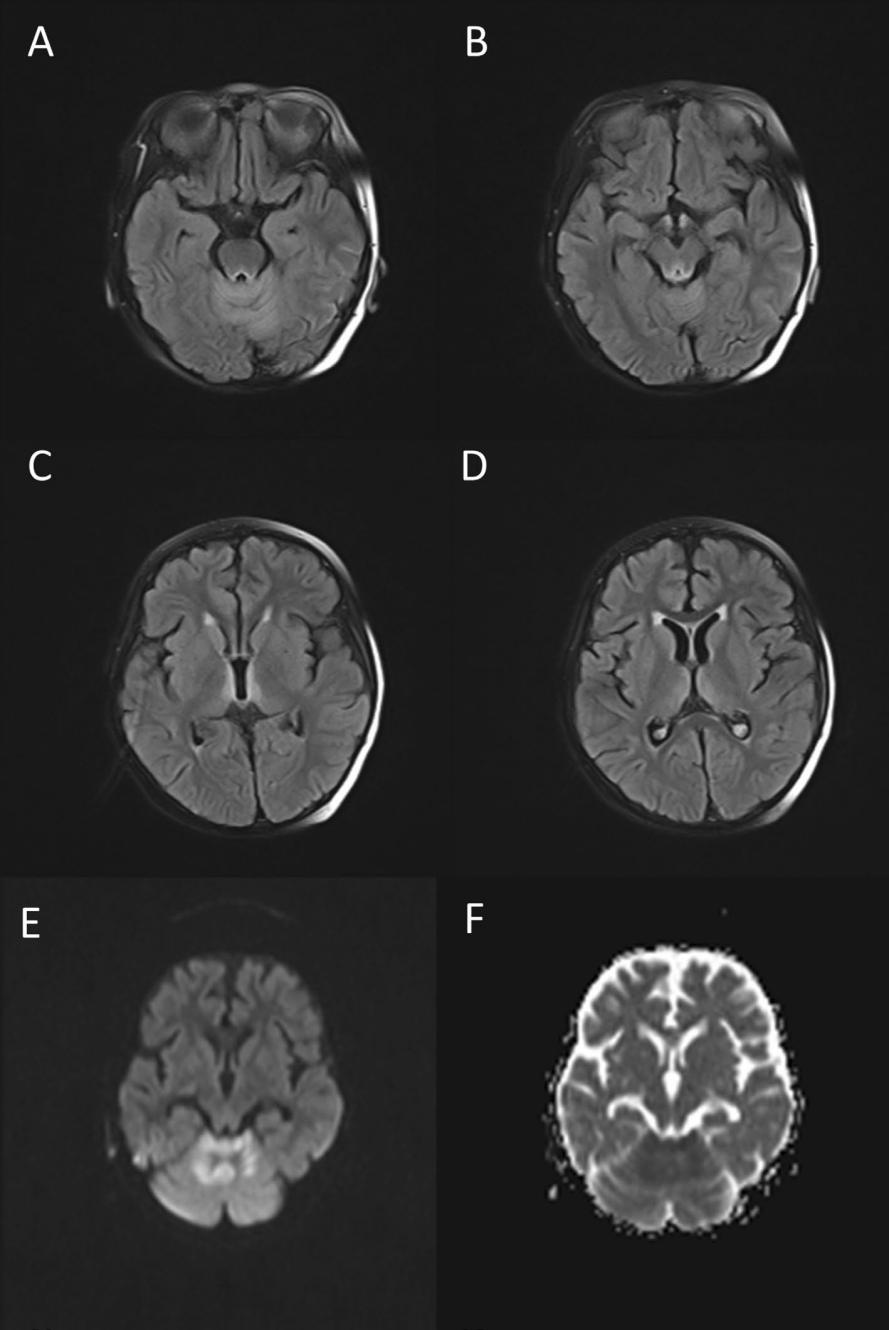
Second MRI: Fluid‐attenuated inversion recovery (FLAIR). MRI showing hyperintensities in the cerebellum, peri‐aqueductal region, and medial thalamic region (A–D). Restricted diffusion more evident in cerebellum (E, F).
